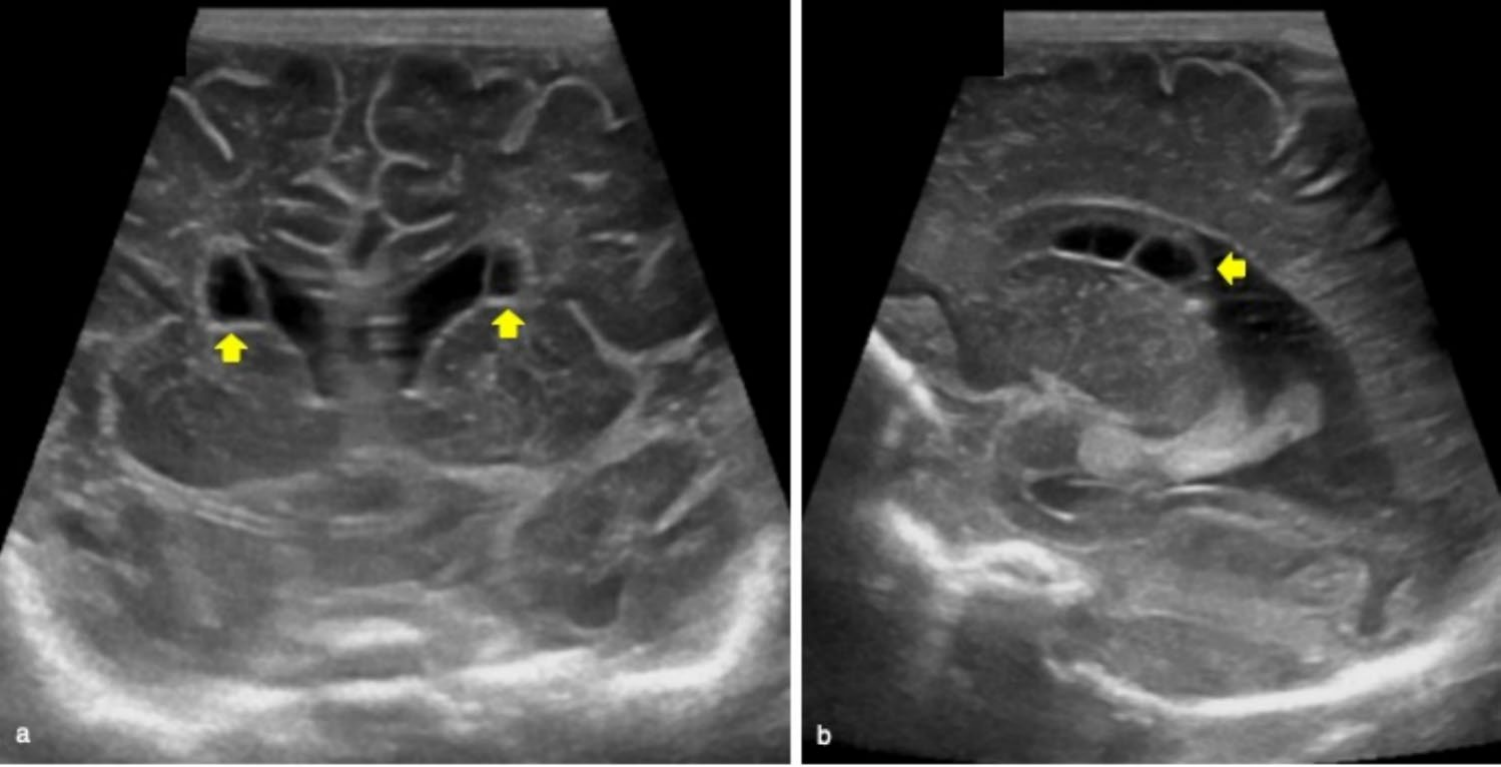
Cerebral ultrasound of 4-day-old full-term male well demonstrating bilateral multiple-rounded cysts located in the frontal horns of the lateral ventricles on coronal plane (yellow arrows in a) with the typical “string-of-pearls” appearance on sagittal view (yellow arrow in b). These findings are suggestive of connatal cysts
